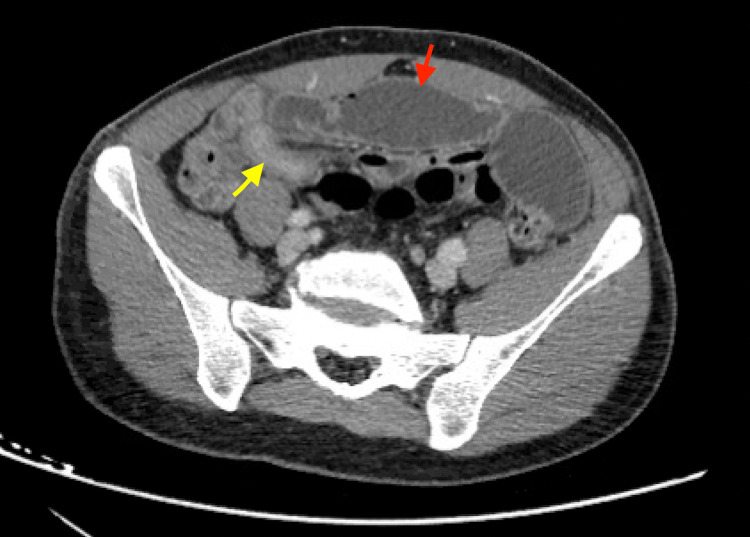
CT abdomen and pelvis demonstrating the thickened segment of ileum (yellow arrow) resulting in the transition point located in the right lower quadrant, with dilation of proximal loops of small bowel (red arrow).
